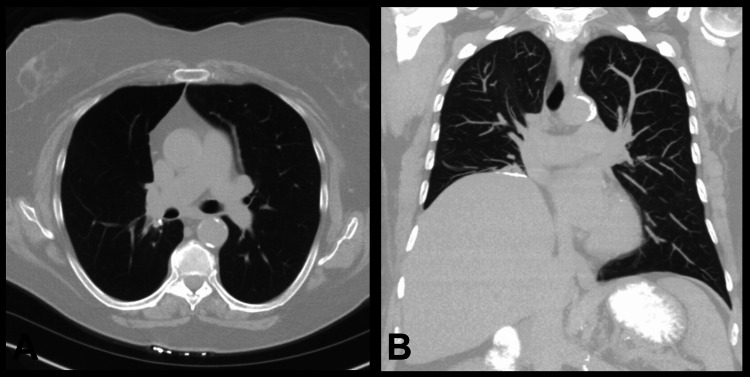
Six-month post-operative computed tomography scan of the chest A computed tomography scan demonstrating an axial (A) and coronal (B) view of the patient’s lungs following resection of the right lower lobe. Normal post-operative changes are demonstrated and no recurrence of the tumor is visualized.
